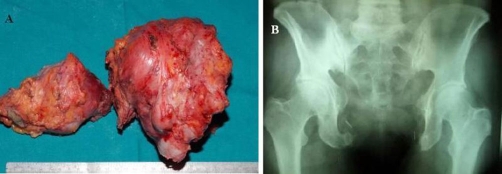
The removed chondrosarcoma (A) and a two-year-postoperative radiograph (B).
